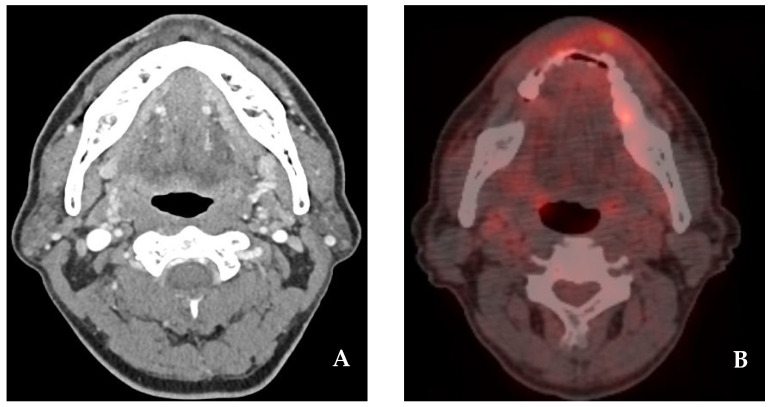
(A) CT neck(e). The image enhances the mass lesion with a size of 3.0 cm × 1.0 cm on the lower lip. (B) PET-CT. Focal metabolic lesion on the lower lip.
